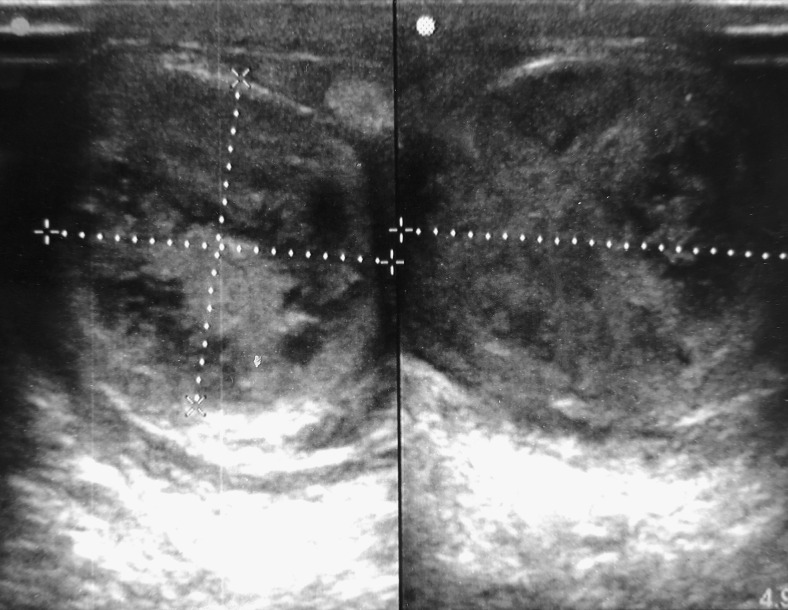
Ultrasound image showing intratesticular hypoechoic areas with mottled hyperechogenicity.
